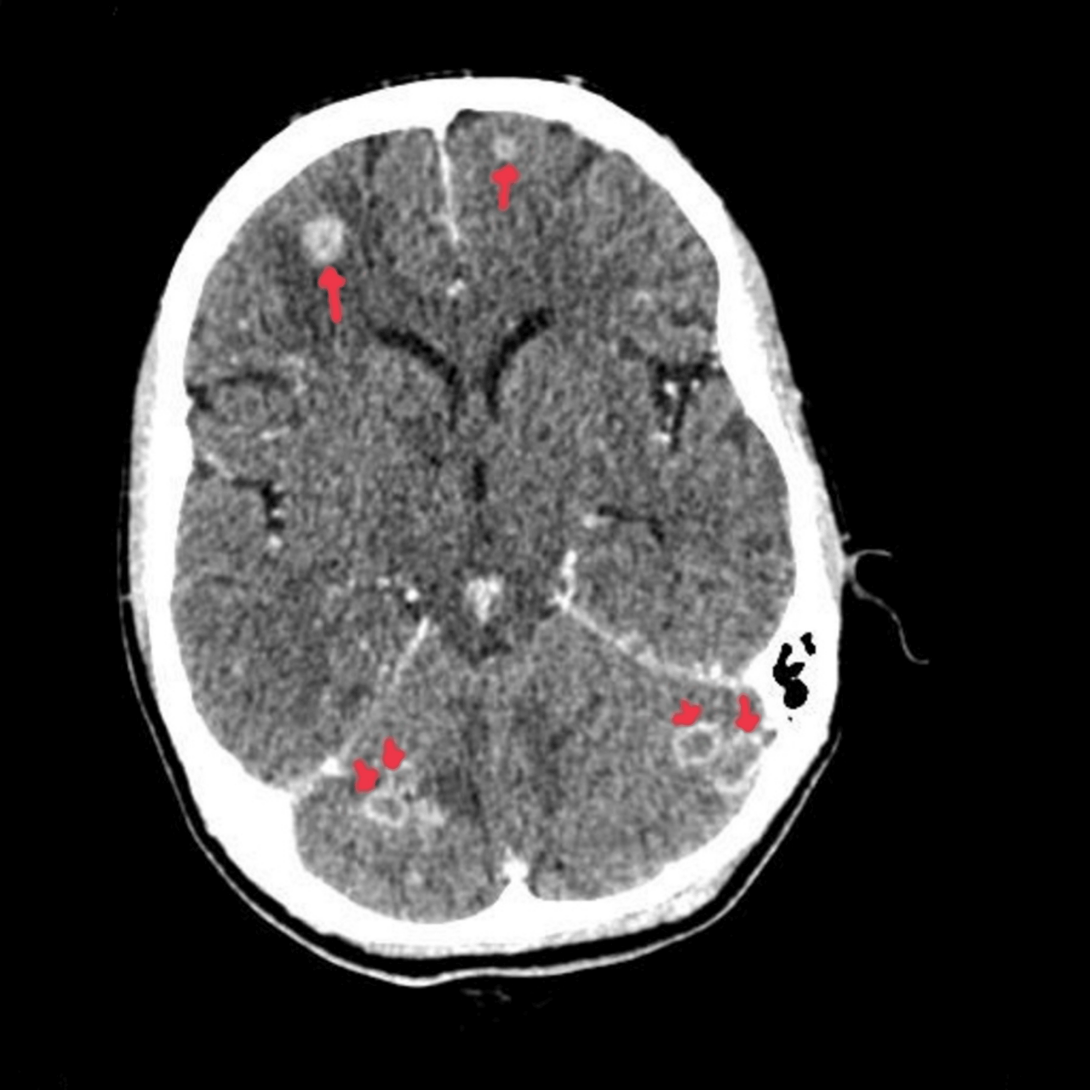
Contrast-enhanced CT head showing ring-enhancing lesions within the cerebellum.
